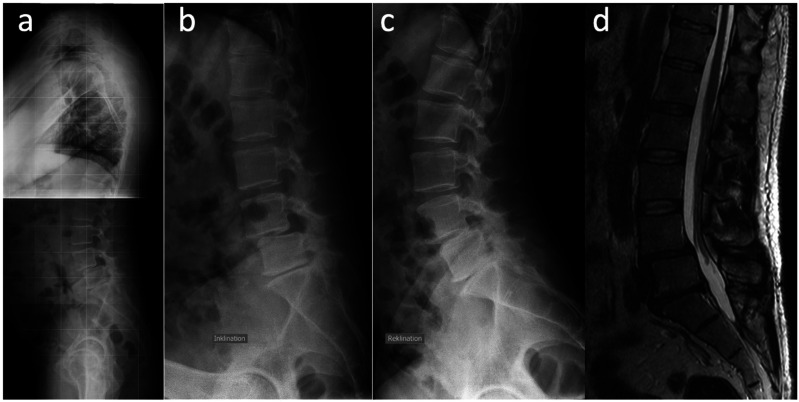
Images of a 48-year-old male patient with a degenerative spondylolisthesis in L4/5 Meyerding II. (a) radiography in sagittal plane of the full spine (SP) in a standing position, (b) flexion (FRF), (c) extension radiography (FRE) in a standing position, and (d) T2-weighted MRI in a recumbent supine, psoas-relaxed position.
